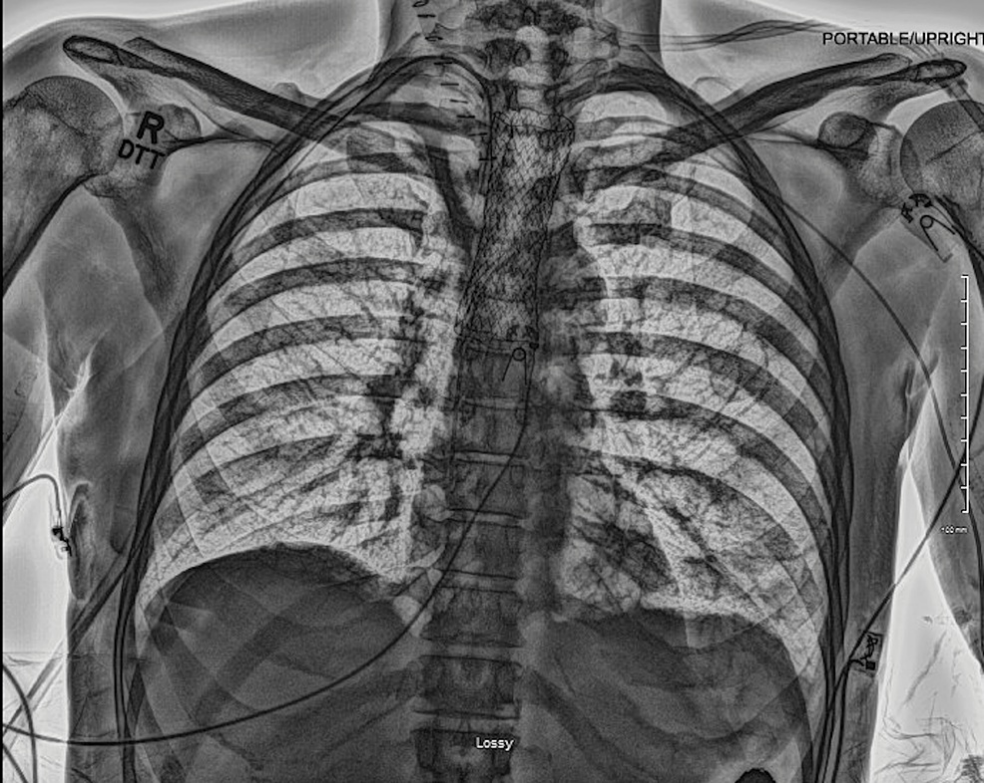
Chest X-ray showing a well-placed esophageal stent
